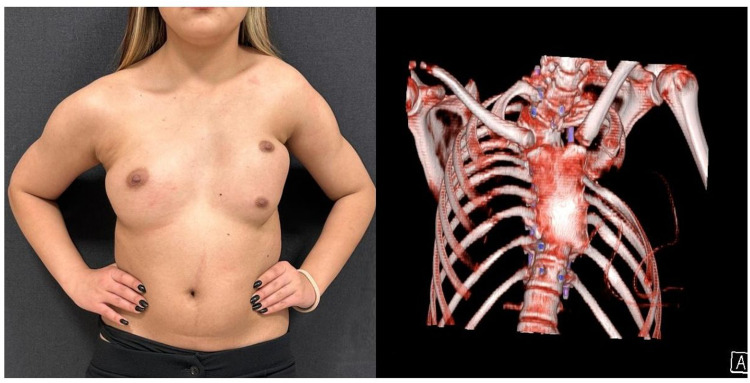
Preoperative patient picture and Preoperative 3D-CT image anterior view that shows rib agenesis.
